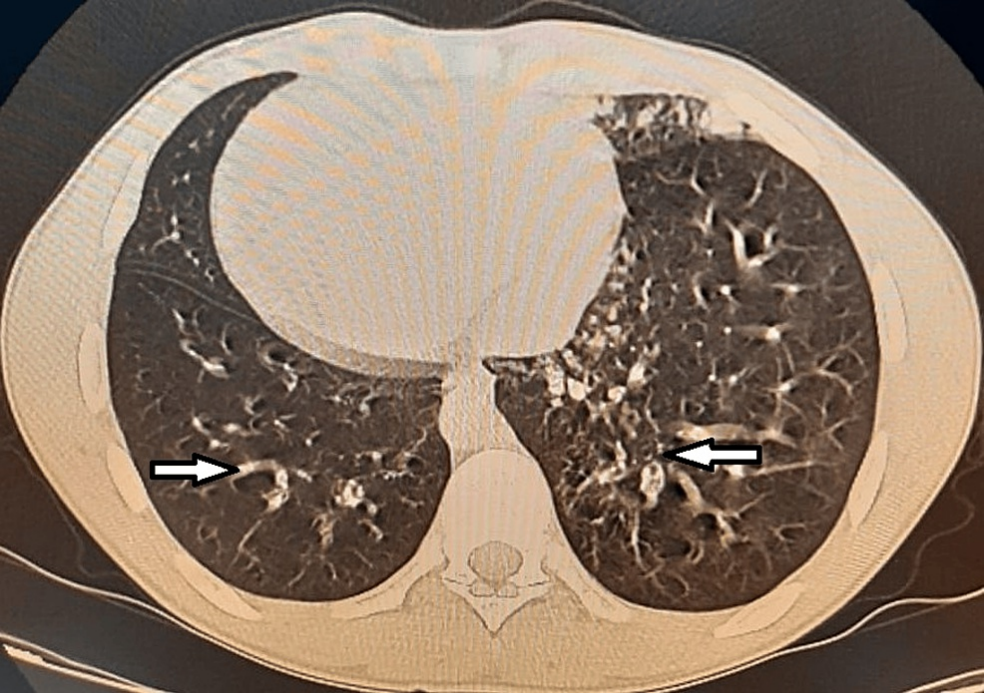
Chest CT scan (parenchymal window) showing the bilateral bronchial dilatation (white arrows) associated with situs inversus
